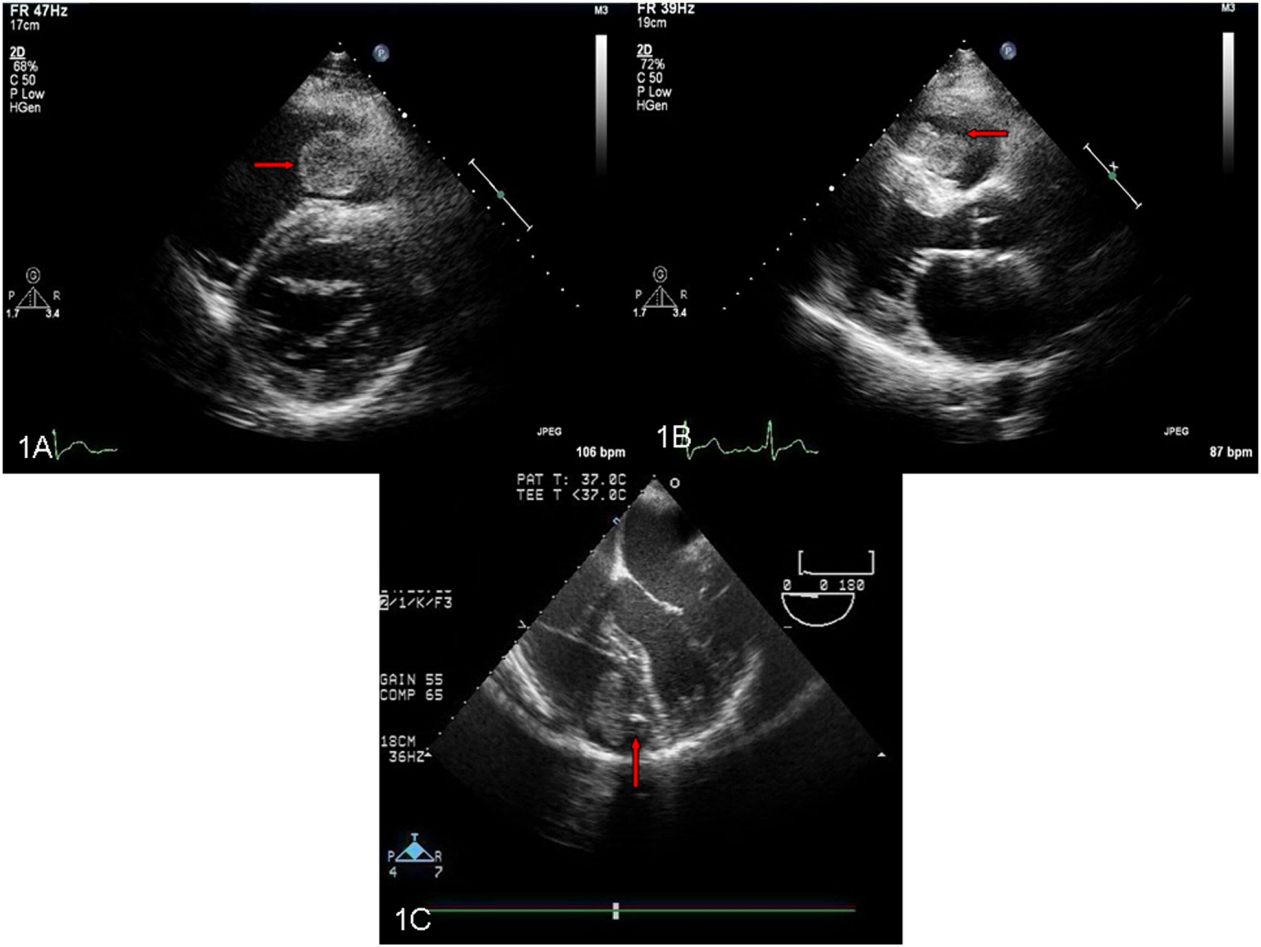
Transthoracic and transesophageal echocardiograms. (A, B) Parasternal long and short axis views showing right ventricular mass (marked with red arrow). C: Transesophageal echocardiogram: Five chamber view, showing right ventricular mass attached with a stalk to interventricular septum (red arrow).
